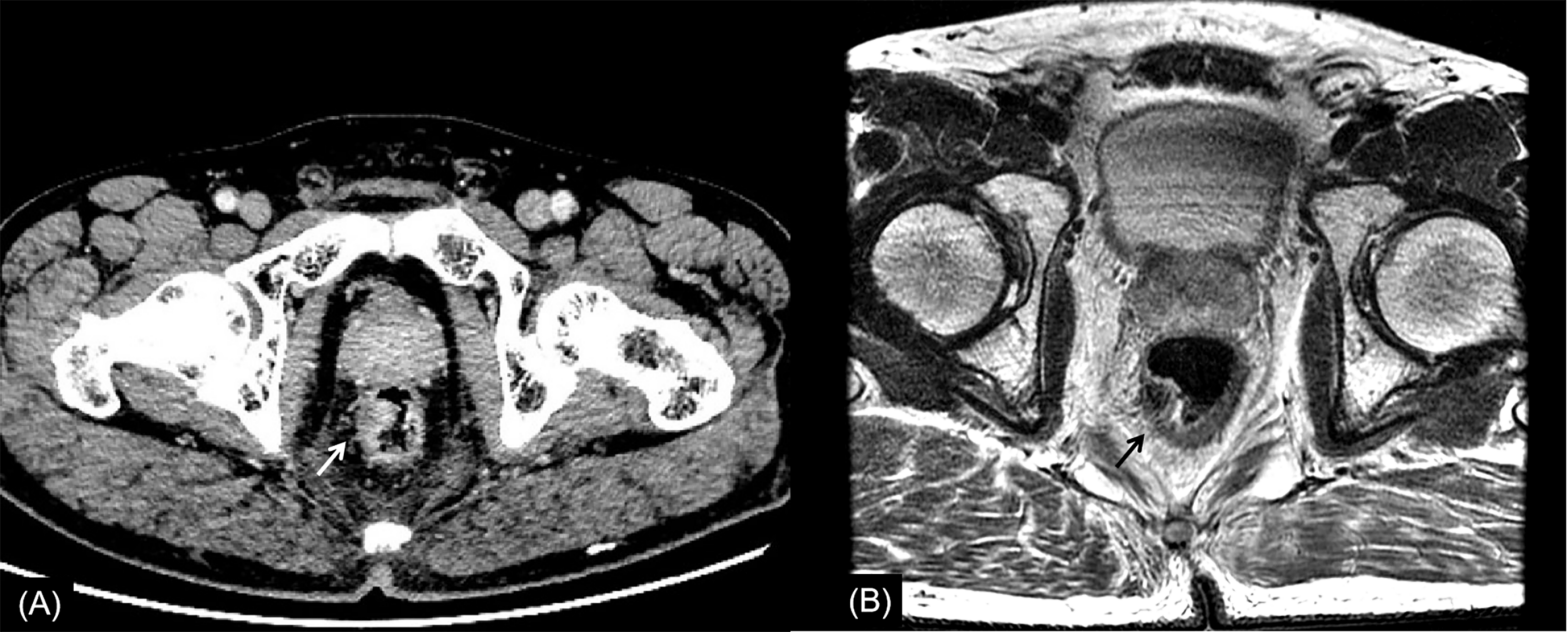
Rectal cancer with ypT1N0. (A) Axial MSCT depicts a mass protruding into the enteric cavity (arrow) and penetrating the muscular layer. Over restaged as T2. (B) MRI transverse high-resolution T2WI of rectum shows a hypointense mass involving muscular layer (arrow). Over restaged as T2. yp: pathological staging after neoadjuvant chemoradiotherapy; T, tumor; N, node; MSCT, multi-slice computed tomography; MRI, magnetic resonance imaging.
